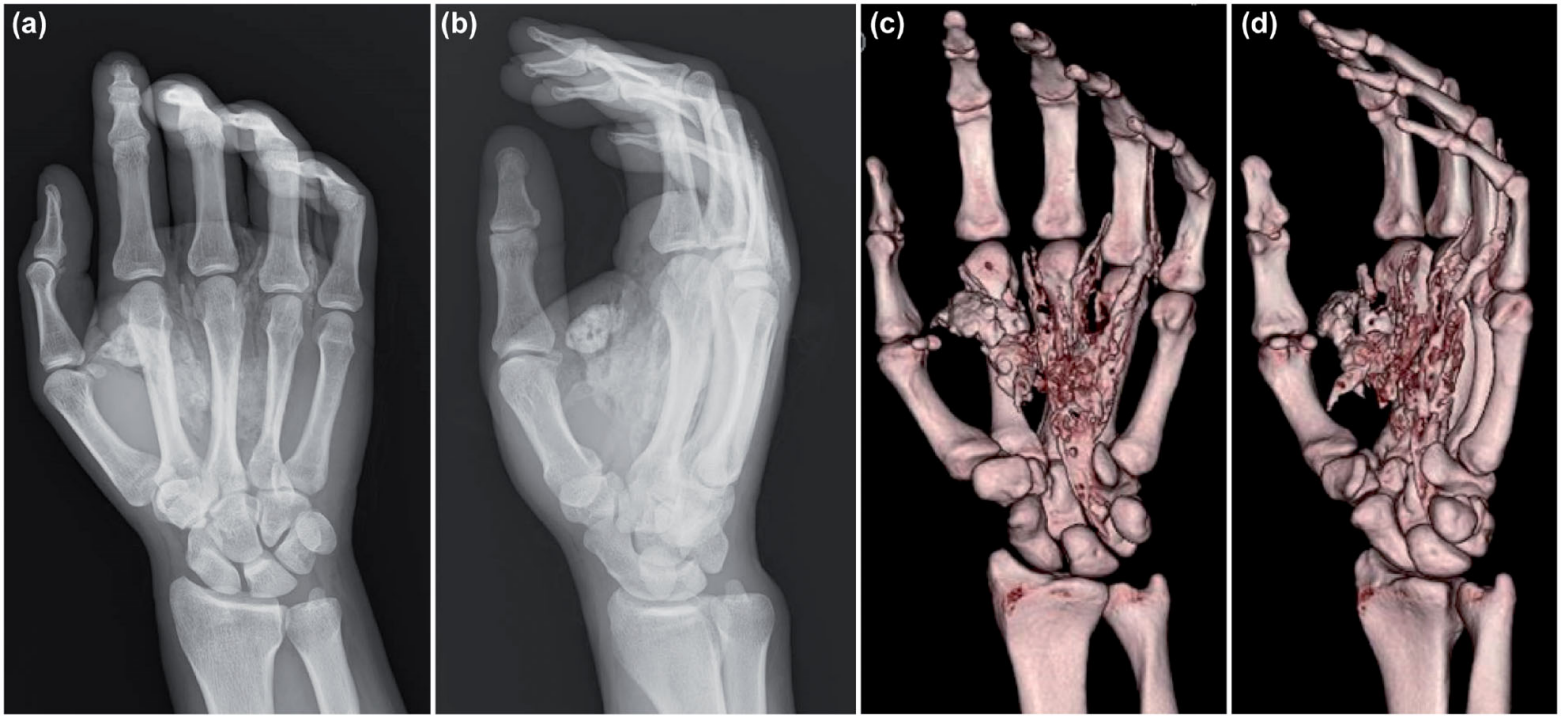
X-ray (a, b) and computed tomography (c, d) images show that the paint spread extensively along the flexor tendons of the palm.
